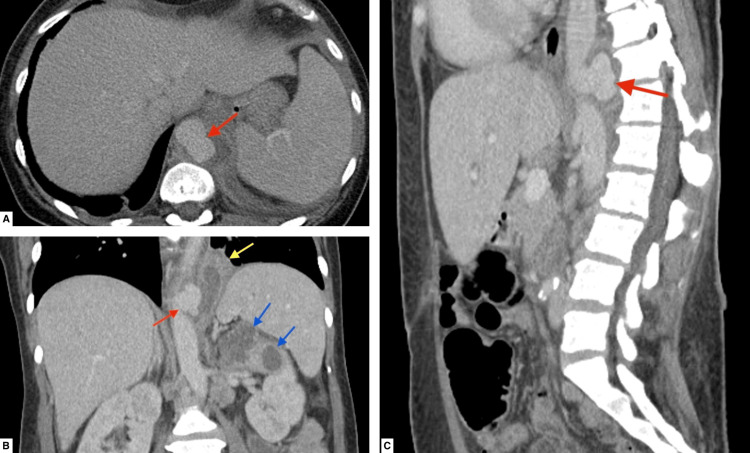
CT scans of abdomen and pelvis with IV contrast showing saccular aneurysm of thoracoabdominal aorta (red arrows in A-C), complex fluid in the left pleural space tracking into the major fissure (yellow arrow in B), and left upper renal pole abscesses (blue arrows in B). IV, intravenous; CT, computed tomography
